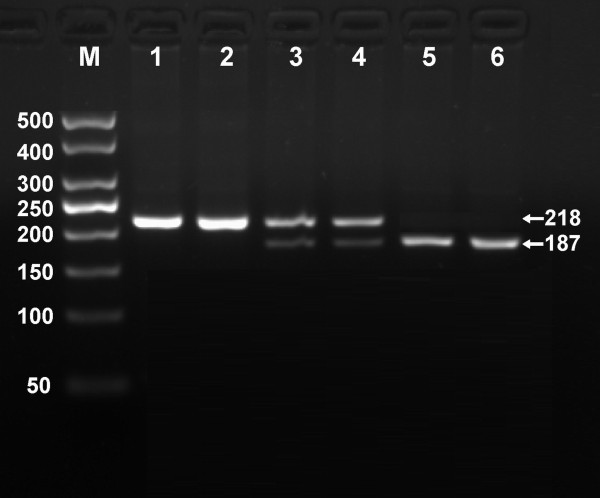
Genotyping of rs5888 SNP in the SCARB1 gene. Lane M, 50 bp marker ladder; lanes 1–2, TT genotype (218 bp); lanes 3 and 4, CT genotype (218-, 187- and 31-bp); and lanes 5 and 6, CC genotype (187- and 31-bp). The 31 bp fragment was invisible in the gel owing to its fast migration speed.
